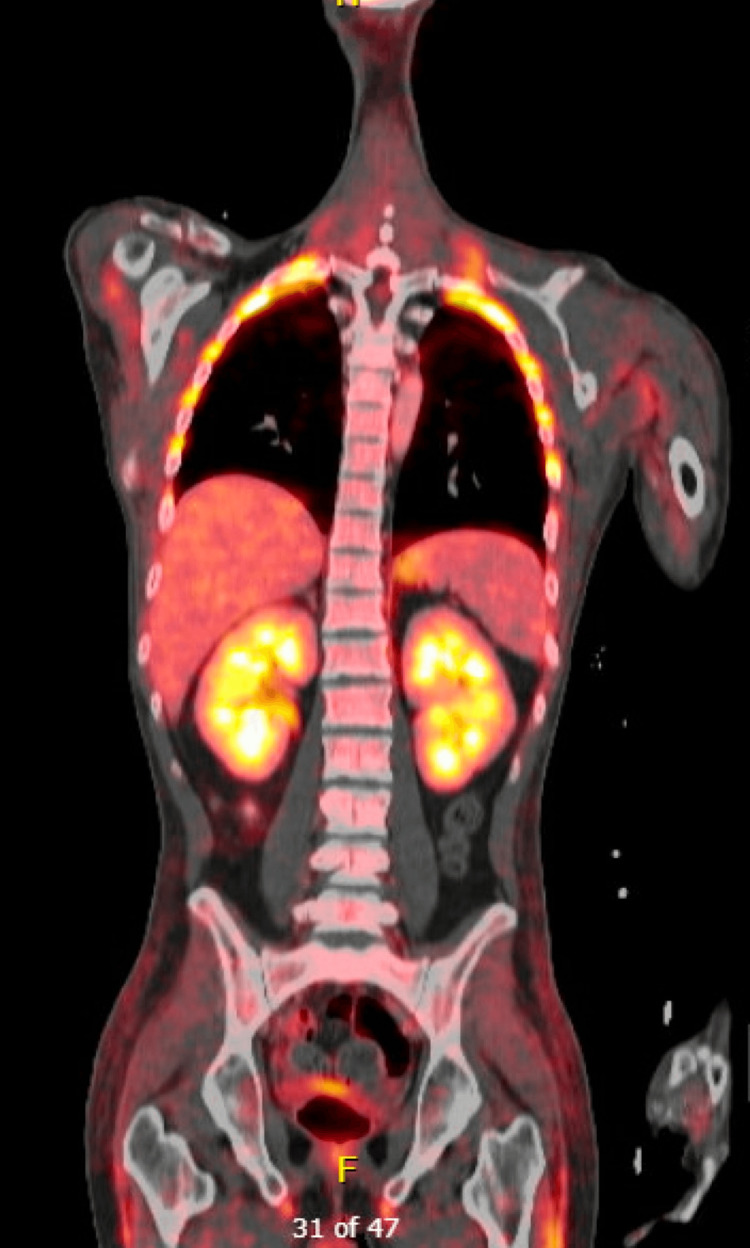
Whole body PET/CT negative for extracranial lymphoma.
